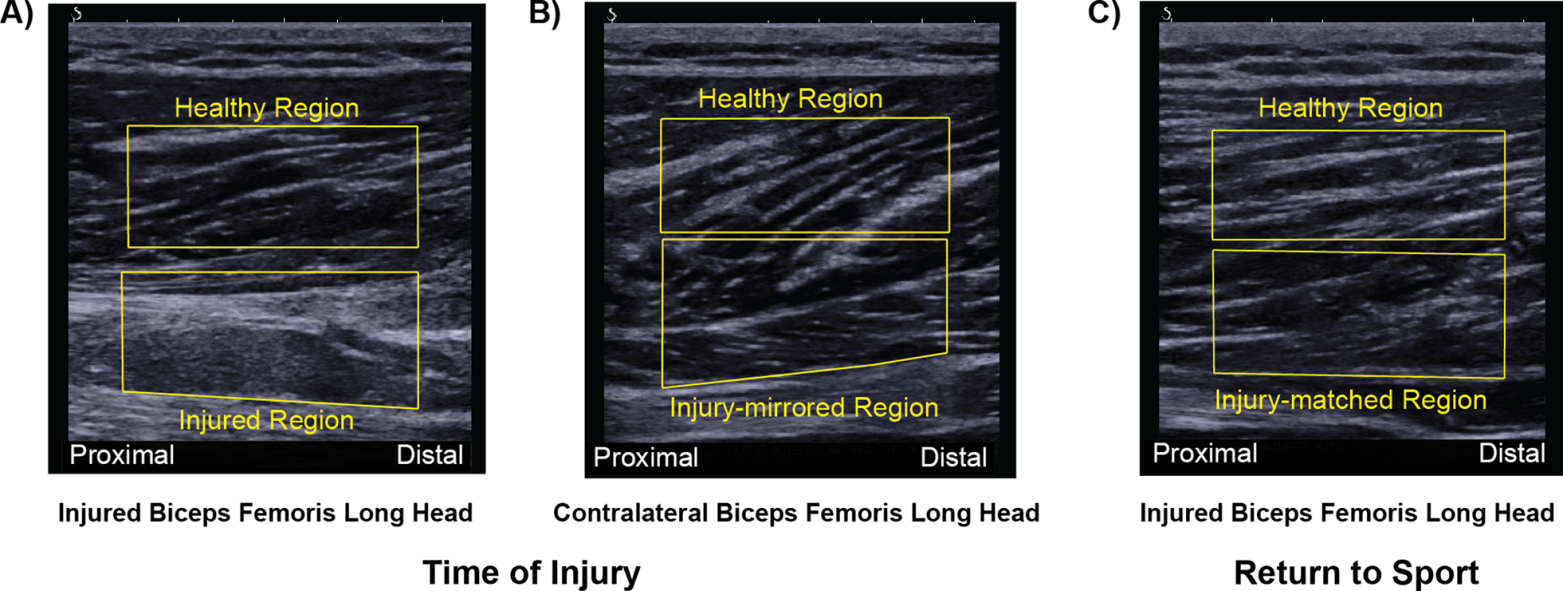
Representative B-mode images from one athlete with regions of interest (ROI) drawn. A Injured biceps femoris long head (BFlh) at time of injury. An ROI was drawn about the region with visually disrupted echotexture or increased echointensity (injured). A second ROI was drawn to capture tissue adjacent to the injured ROI without overlap of the two ROIs (healthy). B Contralateral BFlh at time of injury. The ROIs were drawn to mirror those from the injured BFlh. C Injured BFlh at return to sport (RTS). The ROI was drawn to match those from images obtained at time of injury or to correspond with edema noted on MRI at time of injury if no B-mode images were collected within 7 days of injury
